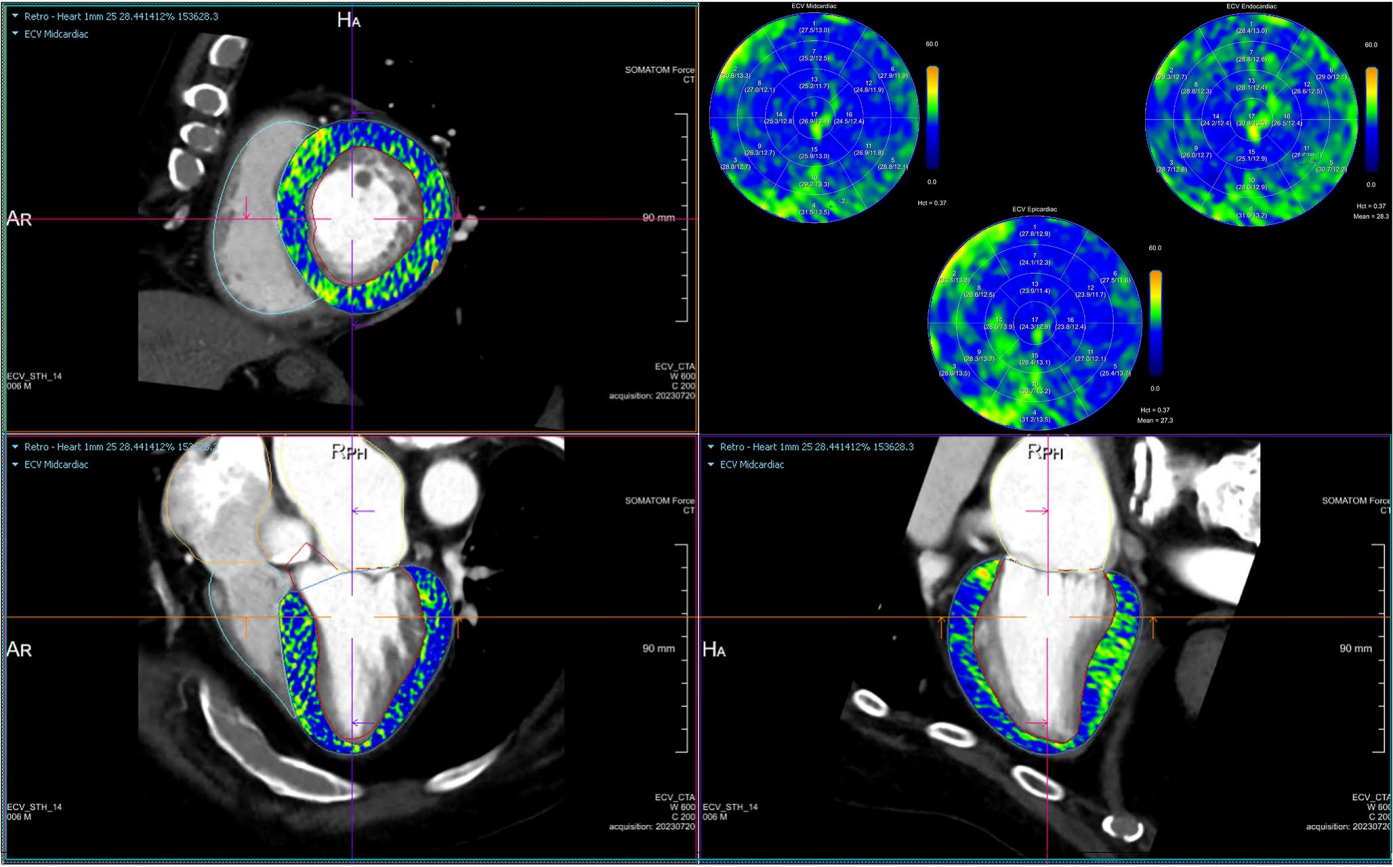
Extracellular volume images and polar maps calculated using the attenuation-based method from late enhancement MDCT images (CT Cardiac Functional Analysis, Siemens Healthcare, Germany). (MDCT: multi-detector computed tomography.)
